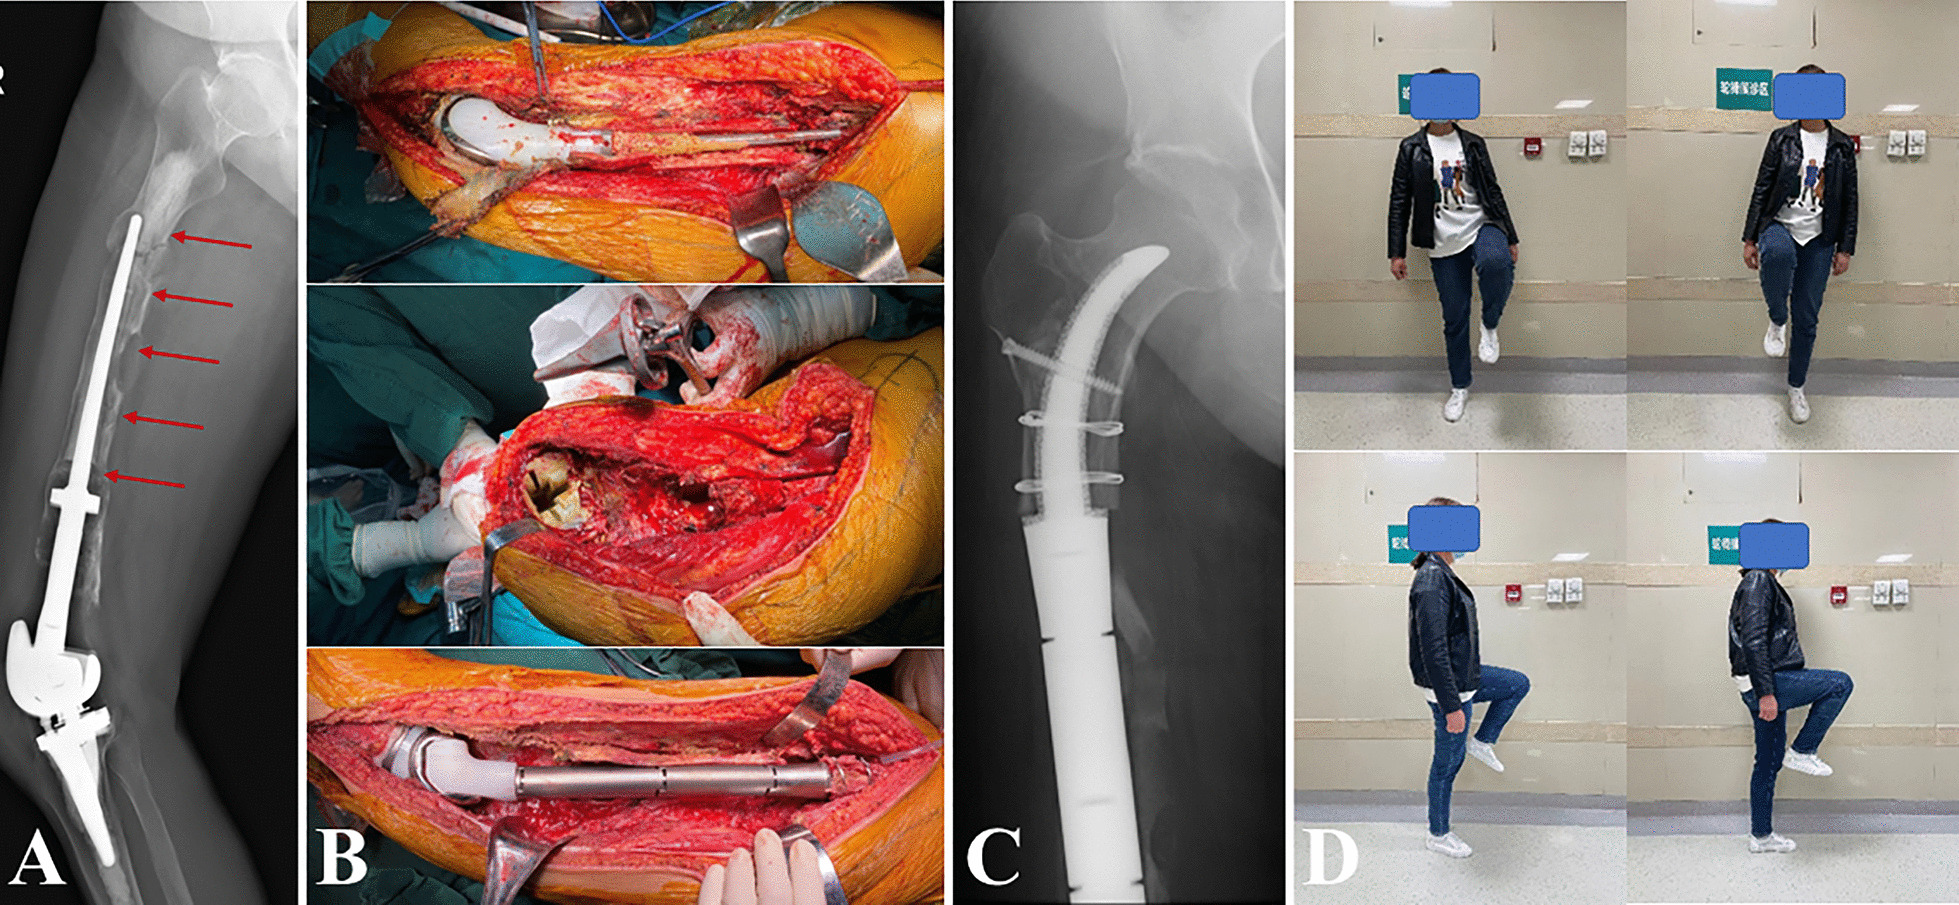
Revision of a Grade IV bone loss case with “Intra-neck curved uncemented stem”. A preoperative radiograph; B intraoperative photographs; C postoperative radiograph 6 months after the revision surgery; D function photographs
